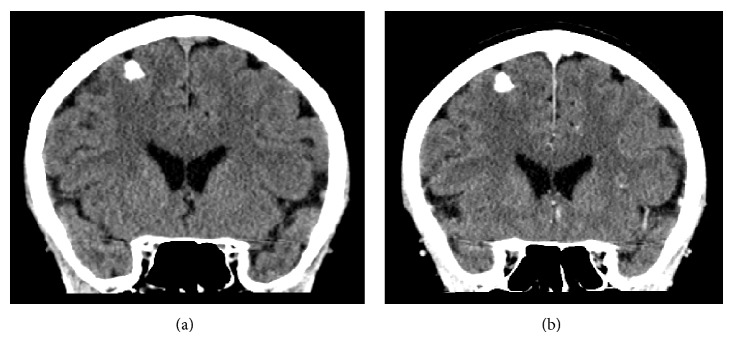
(a) 2013 precontrast CT coronal view. (b) 2013 postcontrast CT coronal view.
